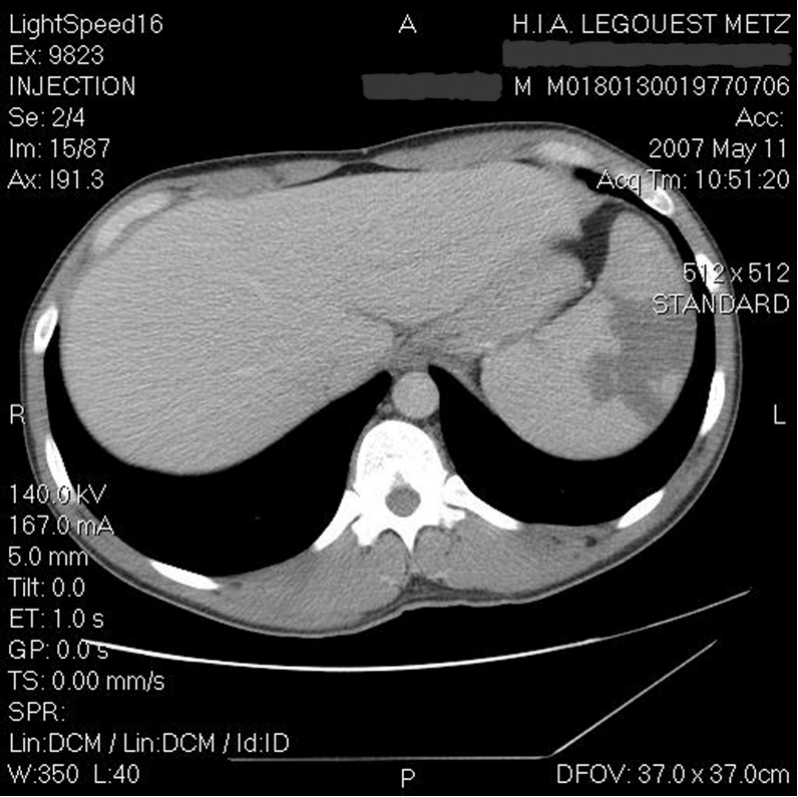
Abdominal CT-scan: splenomegaly and splenic infarction.
